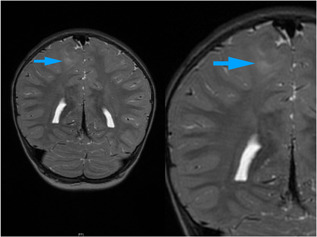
Post‐contrast coronal T2 magnetic resonance imaging with hyperintense signal in the right superior frontal gyrus (blue arrow).
